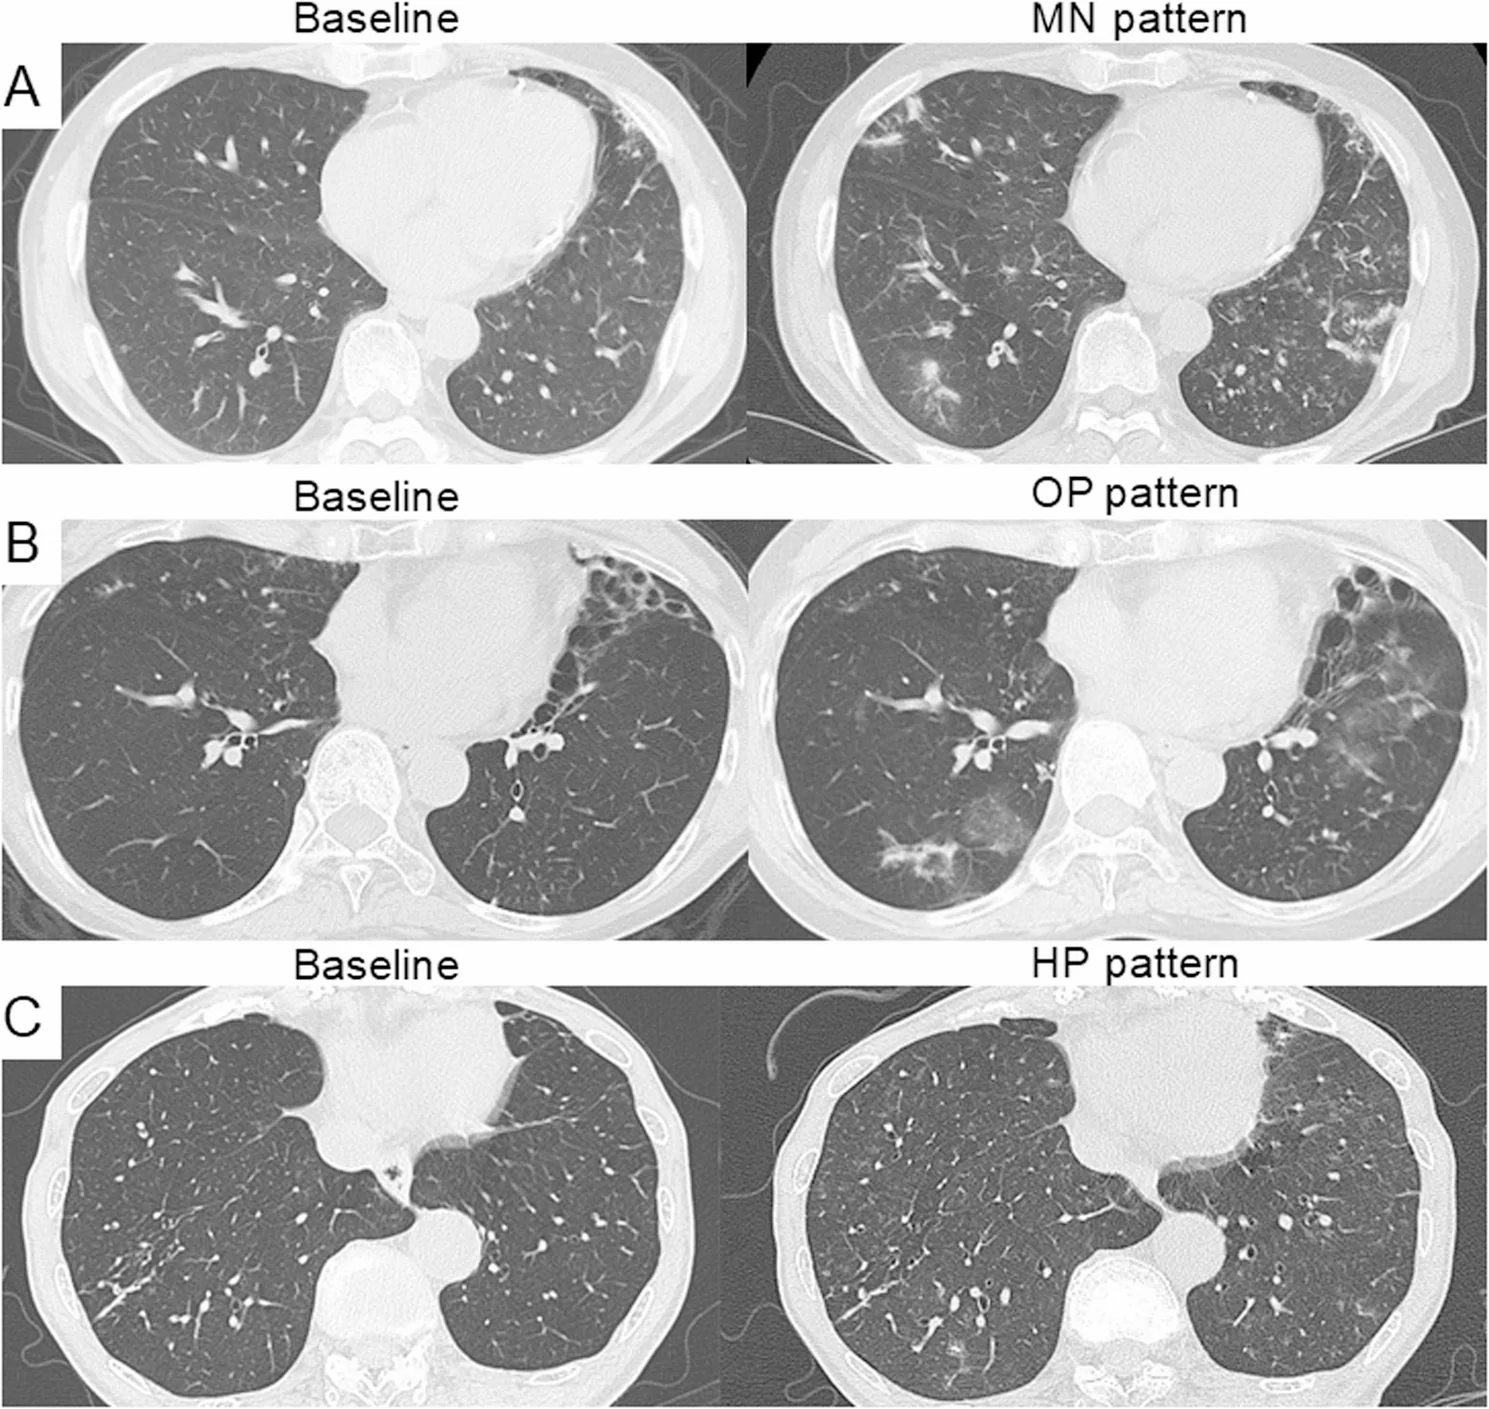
CT findings before ALIS therapy (baseline) and after the development of ALIS-related lung abnormalities. A Baseline (left) and multiple centrilobular nodules (right, MN pattern). B Baseline (left) and patchy ground-glass opacities with a reversed halo sign (right, OP pattern). C Baseline (left) and diffuse ill-defined centrilobular micronodules (right, HP pattern). CT, computed tomography; ALIS, amikacin liposome inhalation suspension; MN, multiple nodular; OP, organizing pneumonia; HP, hypersensitivity pneumonitis
